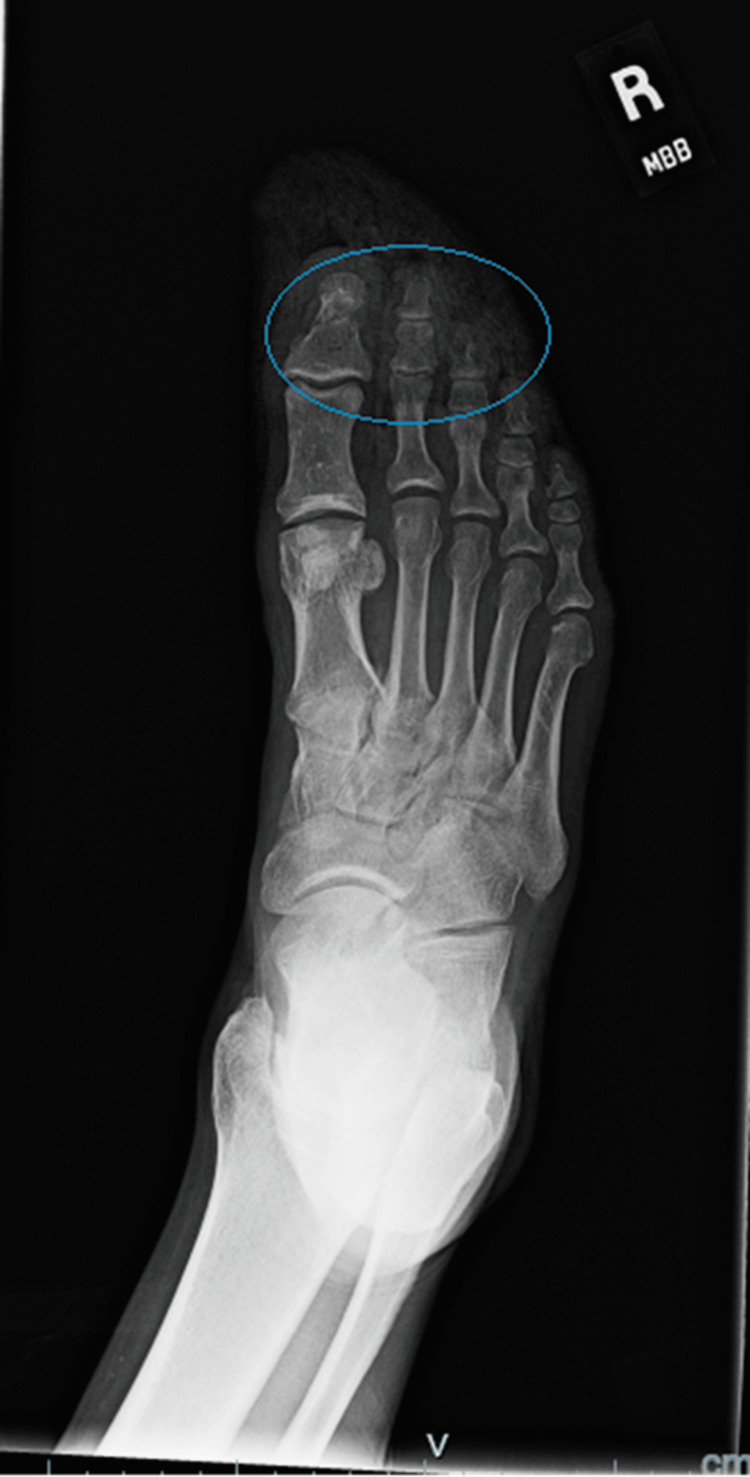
X-ray of the dorsum of the patient's right foot outlining the fractures of all three toes
